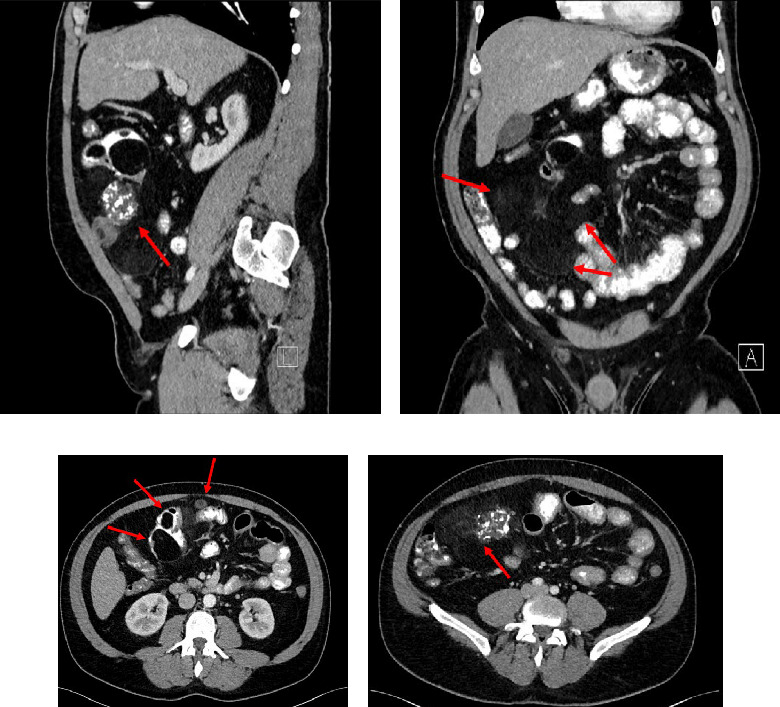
(a–d) CT scan abdomen, mass with both fatty density, and bony components denoted by arrows.
